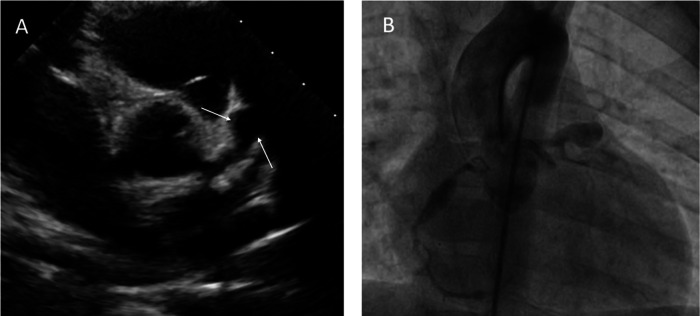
Transthoracic echocardiography showing a giant aneurysm at LAD (A). Coronary angiogram showing multiple giant coronary saccular aneurysm at LAD and RCA (B).
